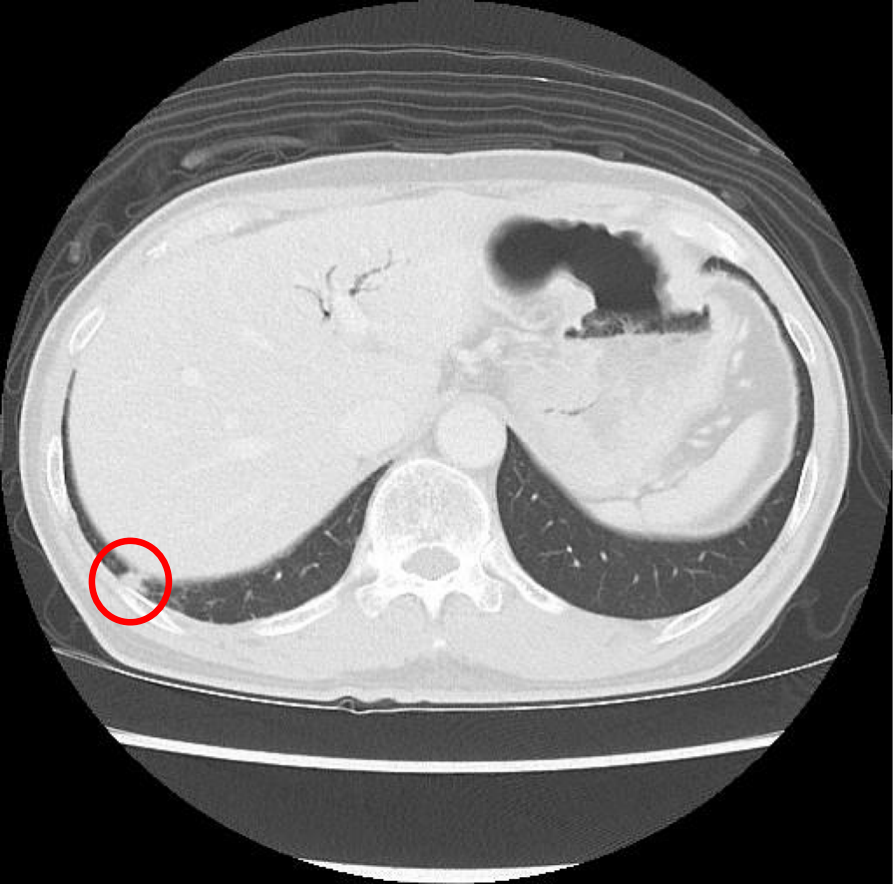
Computed tomography (CT) shows a 5 × 3-mm tumor mass near the base of the right lung. This was a new isolated, localized, solid lesion
